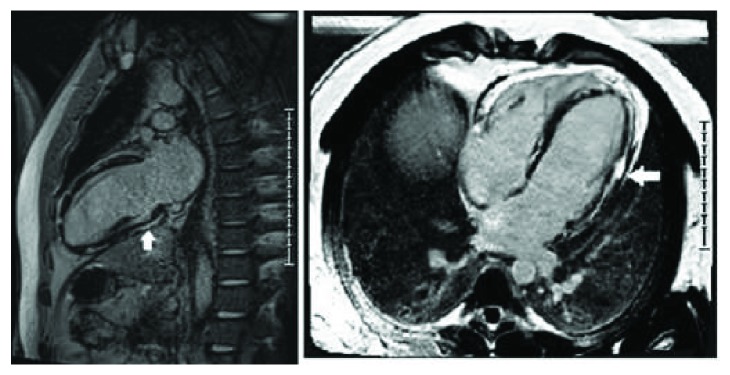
Cardiac MRI showing extensive epicardial and midwall fibrosis in the anterolateral, lateral, inferolateral, and inferior walls from base to apex.
